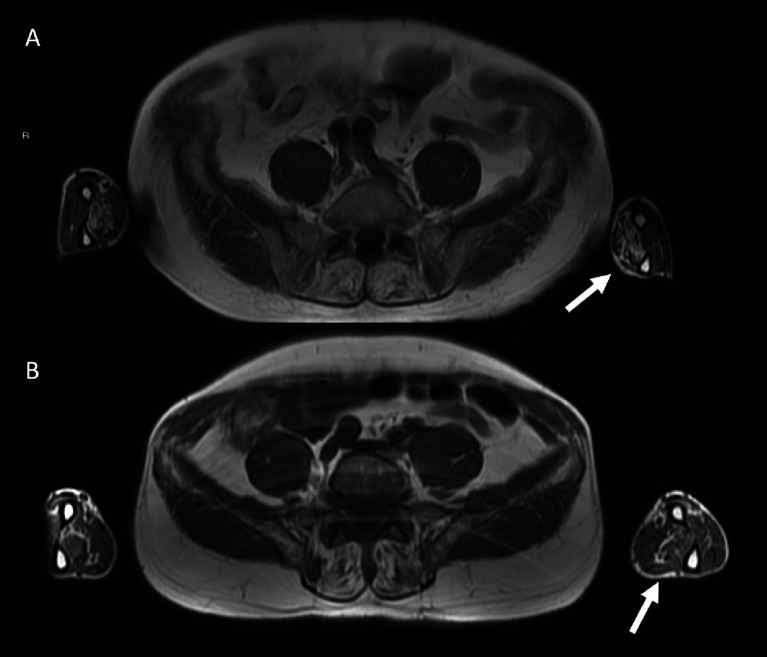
Axial fast spin-echo T1-weighted Whole-Body Muscle Magnetic Resonance Imaging (WBMRI) in a 78 year-old male with inclusion body myositis (IBM) shows moderate-severe fatty infiltration in the flexor digitorum profundus muscles bilaterally (A). A 62 year-old male with inflammatory myopathy with mitochondrial pathology (IM-Mito) shows similar imaging appearance with moderate fatty infiltration in the flexor digitorum profundus muscles bilaterally in axial fast spin-echo T1-weighted WBMRI (B).
